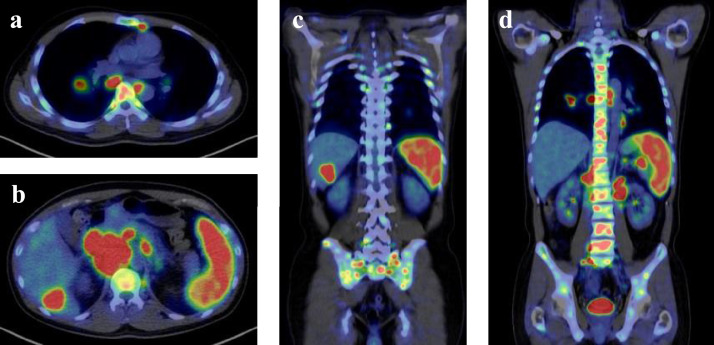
Positron emission tomography-computed tomography demonstrated pathological ^18^F-fluorodeoxyglucose uptake in mediastinal lymph nodes (a, d), abdominal lymph nodes (b, d), spleen (b, c, d), liver mass (b, c), and systemic bone (a, b, c, d).
